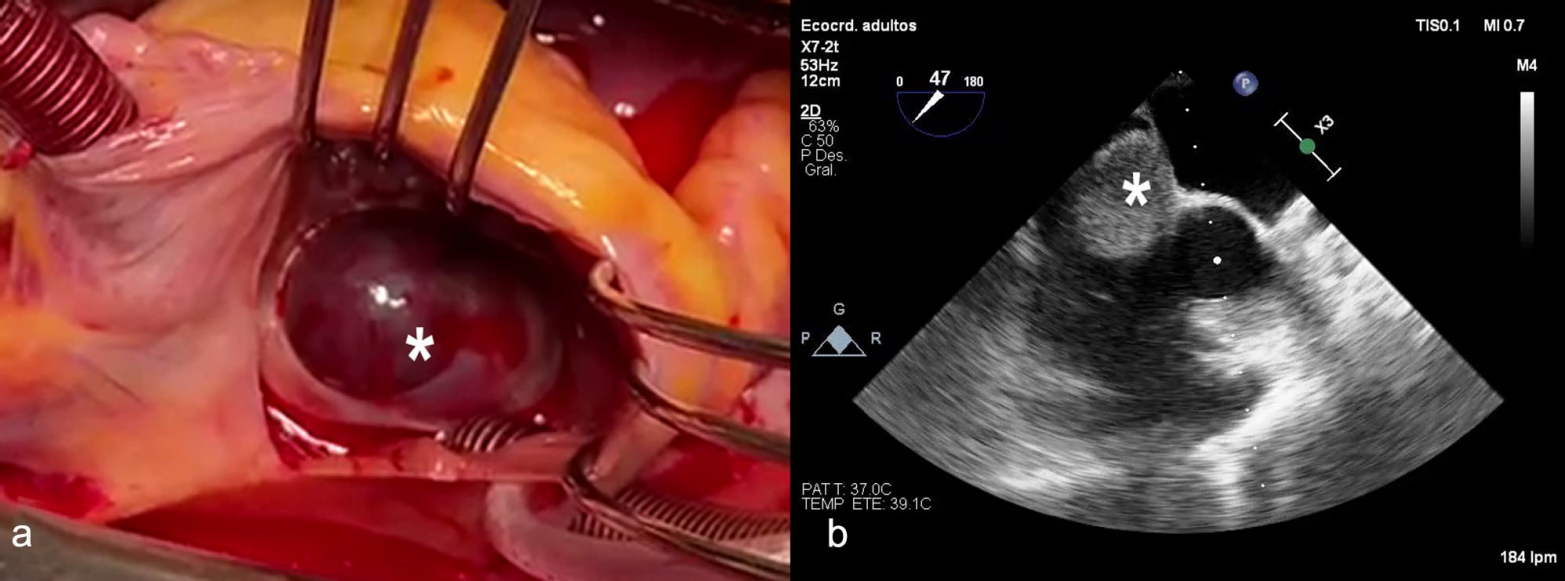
(a) *Intraoperative hemangioma. Surgical vision from the AR. (b) Transthoracic echocardiogram showing the mass in the RA adhered to the IAS
